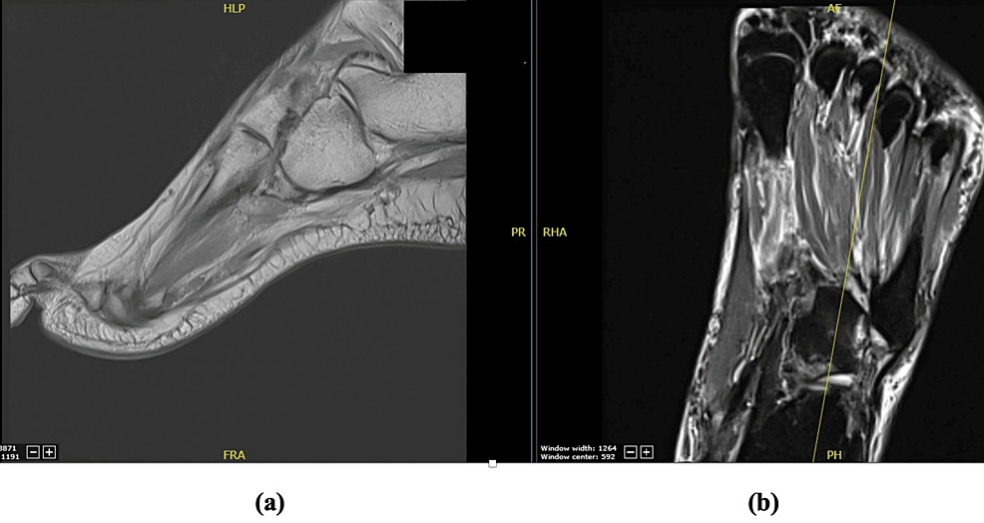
Sagittal (a) and coronal (b) MRI views of the foot pre-operatively demonstrating the inferomedial migration of the cuboid.
